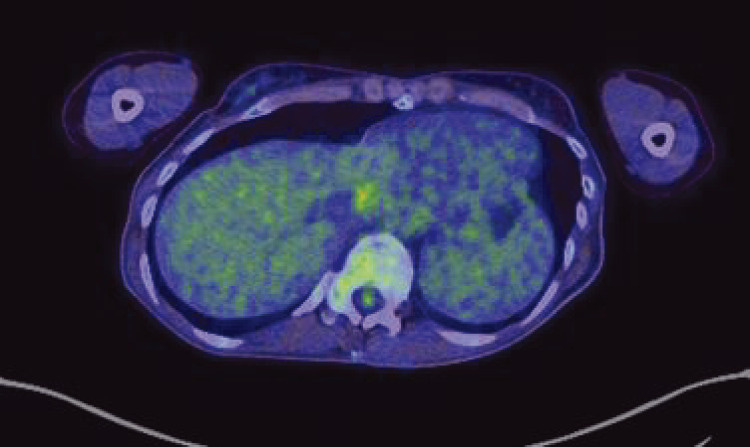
Fig. 4 The tumor showed FDG uptake (e/d SUVmax = 3.02/3.39), indicating significant accumulation. FDG: fluorodeoxyglucose
